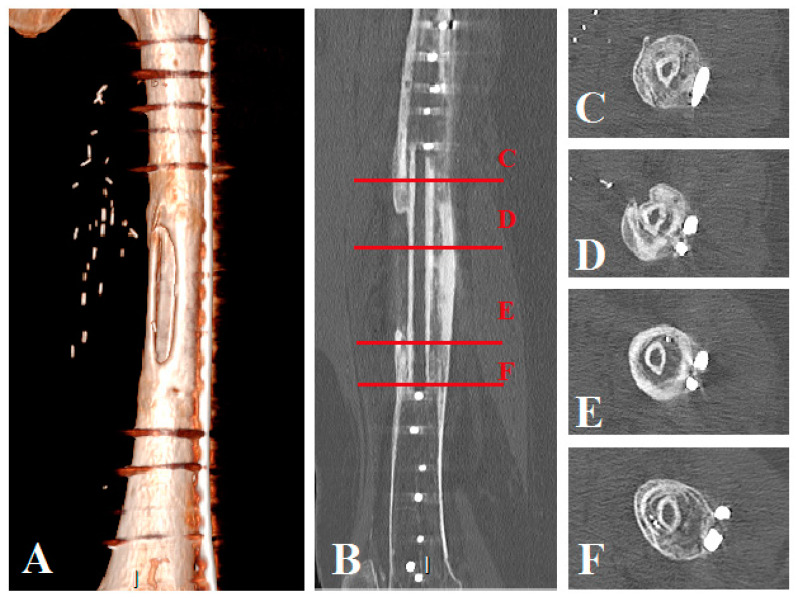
Patient No. 1 CT scan control at 14 months postoperatively: (A) CT scan 3D reconstruction of the healed allograft; (B) sagittal plane view with axial view level representation: (C–F) (C) axial view of the proximal host femur with the healed fibula graft, (D,E) axial views of the healed fibula graft in the allograft, (F) axial view of the distal host femur with the healed fibula graft.
